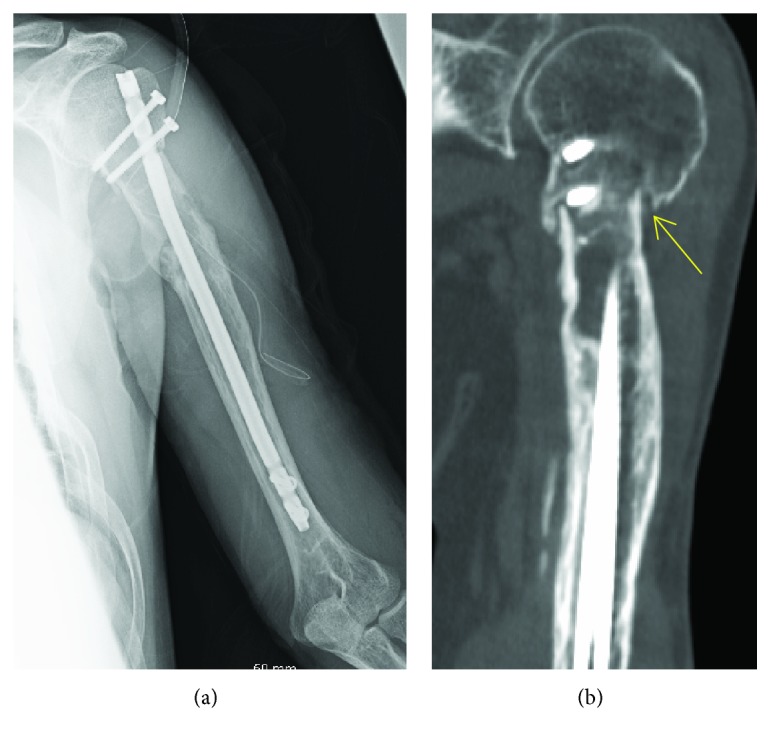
(a) Postoperative radiograph of the left humerus representing internal fixation of the osteotomy site after correction of varus deformity using an intramedullary nail. (b) Postoperative CT at 8 months revealing bone union at the osteotomy site.
